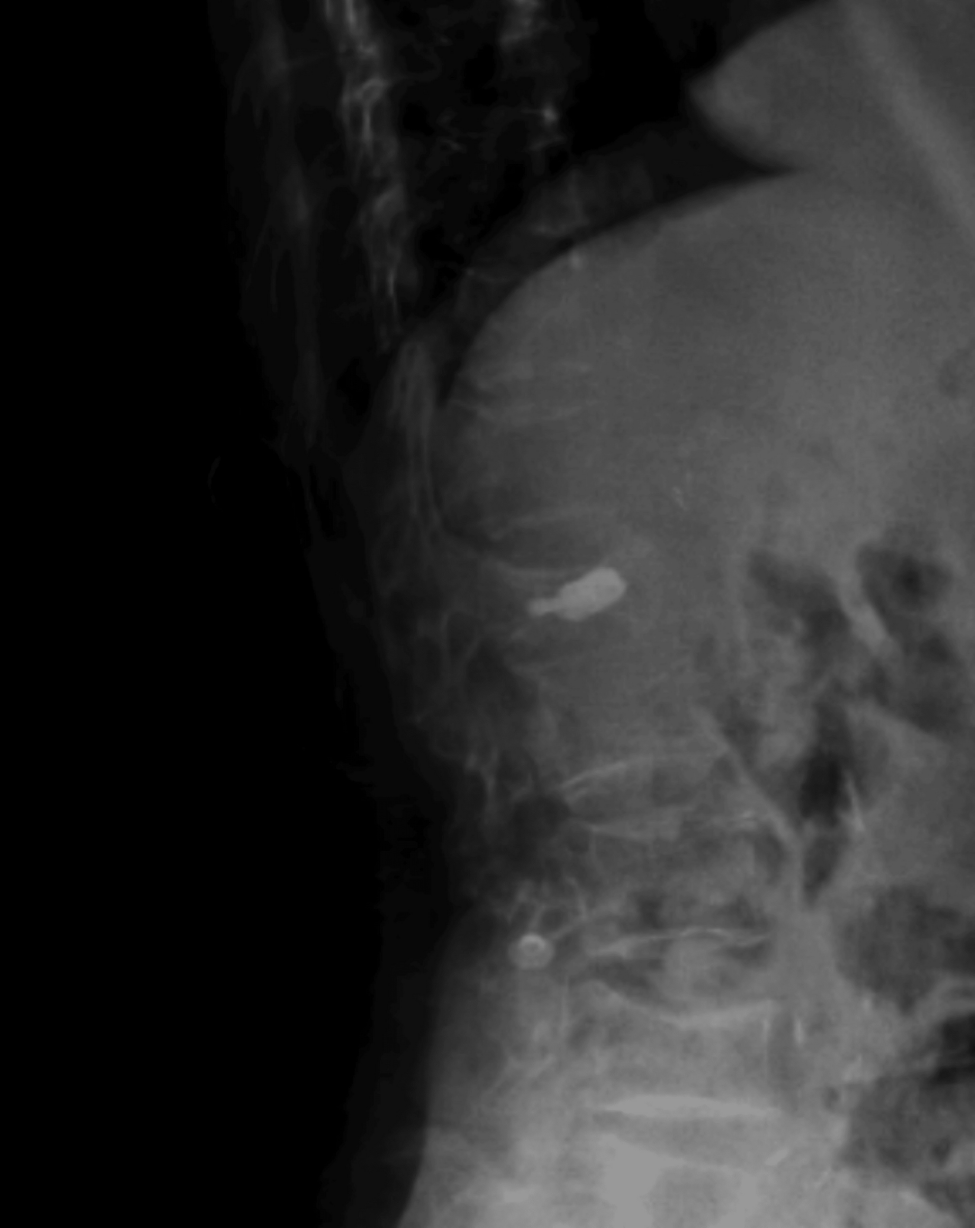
Lateral radiographs showing adequate filling of bone cement within Vessel-X in the L1 vertebral body resulting an increase in its vertical height
